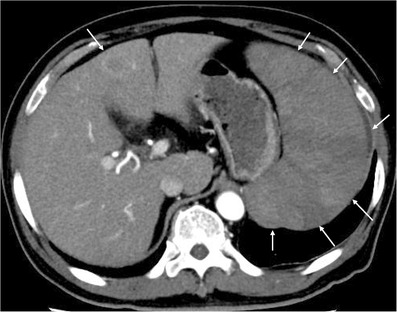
Contrast‐enhanced abdominal computed tomography (CT) reveals massive splenomegaly and a space‐occupying lesion with ring‐like enhancement in the S4 segment of the liver
